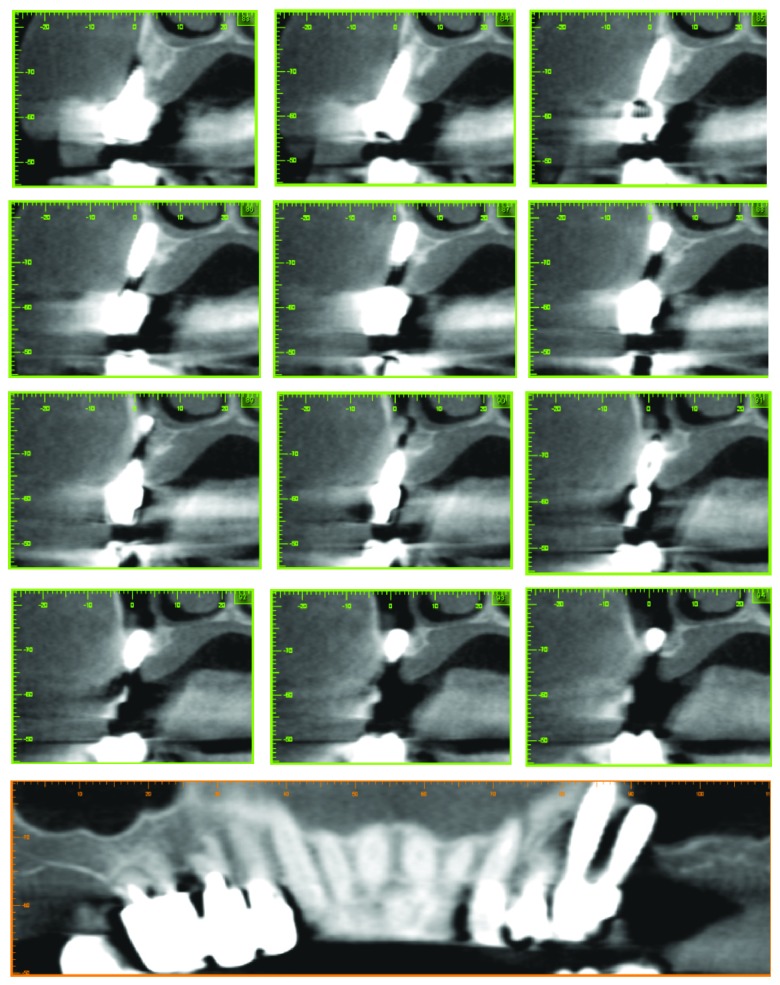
CBCT scans images showing the large concave bone resorption due to peri-implant diseases involving implant-supported rehabilitation of 2.5 and 2.6.
